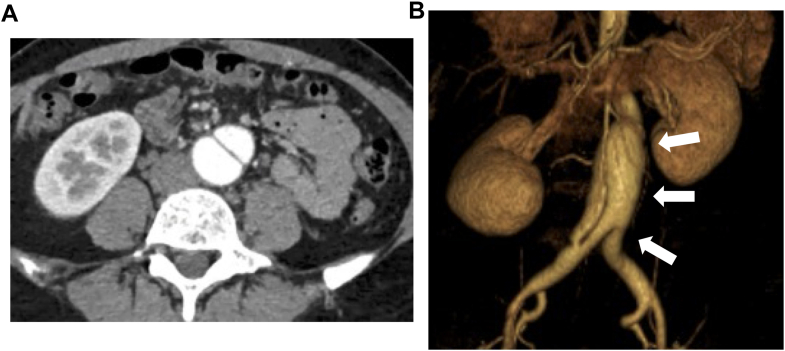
Incidental Infrarenal AAA and Dissection (A and B) Computed tomography scan showed 37 mm of infrarenal abdominal aortic aneurysm (AAA) complicated by dissection extending to the iliac artery; there was no evidence of atherosclerosis.
